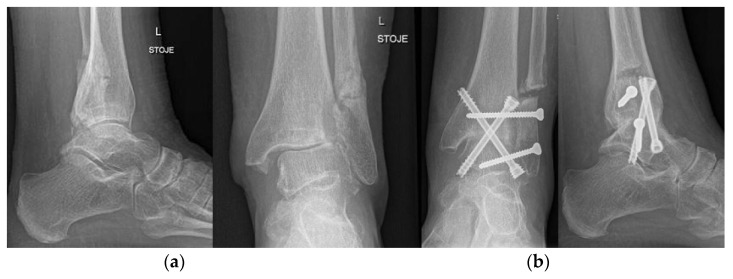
Ankle arthrodesis. (a) preoperative X-ray; (b) postoperative X-ray after ankle arthrodesis.
